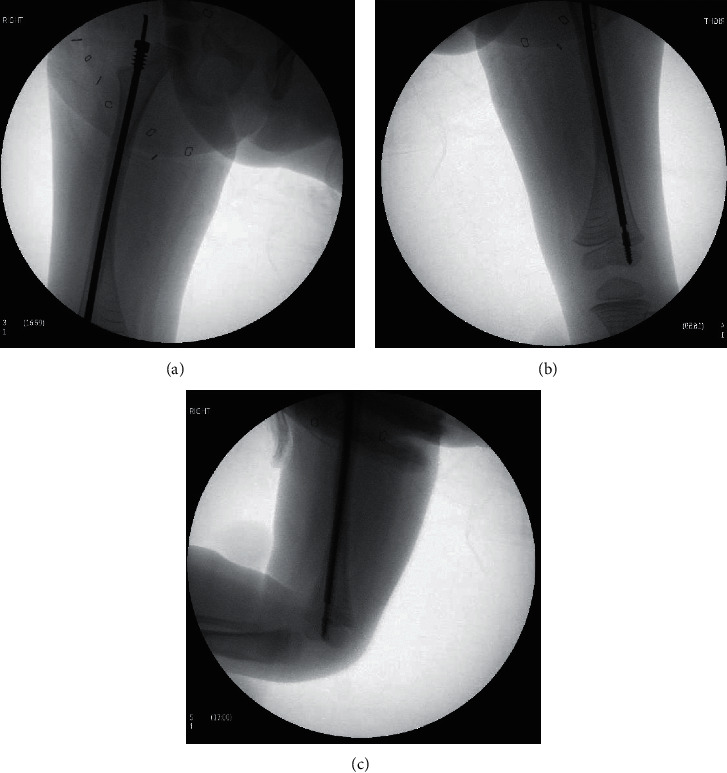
(a)–(c) AP and lateral radiographs of the 4 mm Fassier–Duval rod in place following removal of the fractured 3.2 mm male retrieval shaft and rod.
